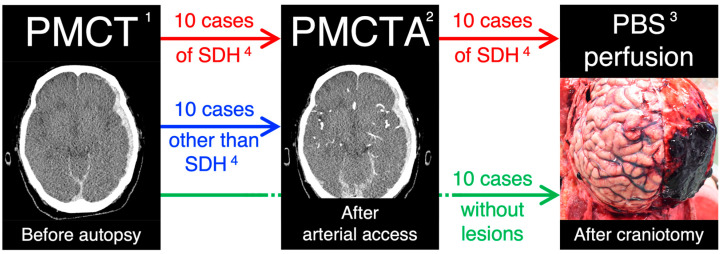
The outline of the methods: (1) postmortem computed tomography, (2) PMCT angiography, (3) phosphate-buffered saline, and (4) subdural hematoma.
